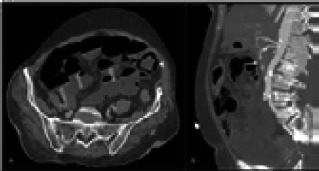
70-year-female COVID positive with abdominal pain and distension. Lab parameters showed raised TLC, CRP & D-dimer. (A) Axial post-contrast CT shows dilated bowel loops with multiple air-fluid levels (white arrows) and presence of intramural air (arrow head) suggestive of pneumatosis intestinalis. (B) Sagittal image in arterial phase shows non opacification of SMA (white arrow) suggestive of thrombosis
